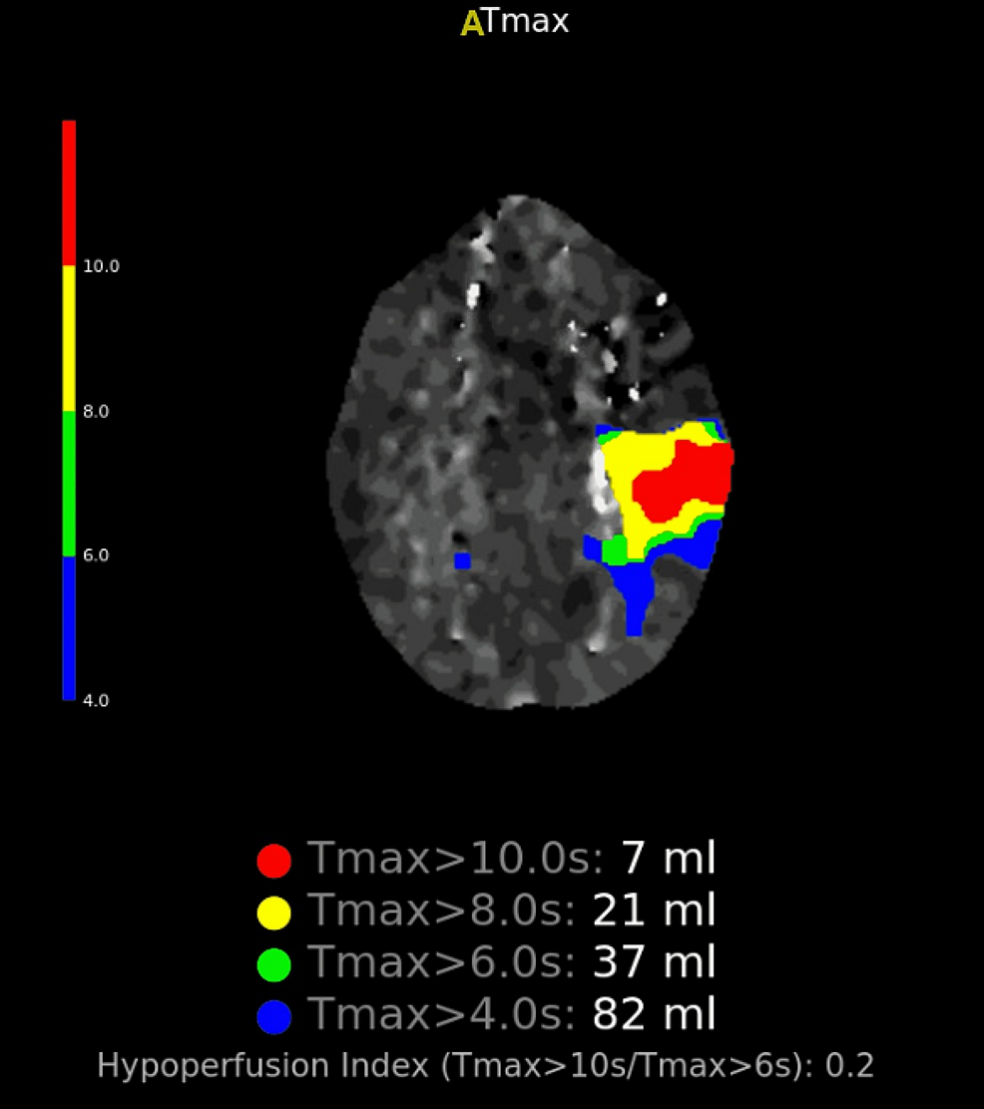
CT perfusion with contrast suggestive of acute ischemia in the left temporoparietal region. No evidence of ischemic infarct core.
